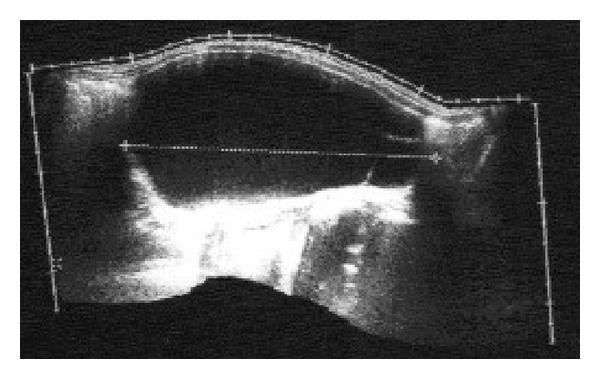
Abdominal ultrasound showing a 14 cm by 7 cm by 12 cm fluid filled structure in the left half of the abdomen.
